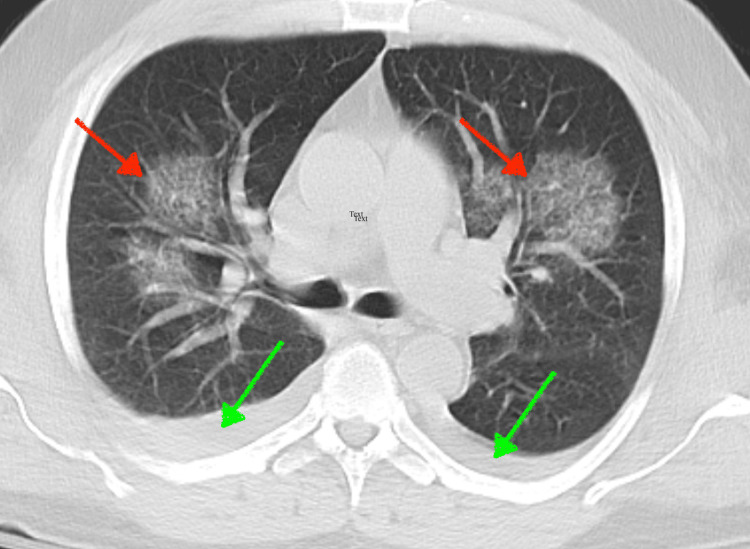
CT chest Lungs: Patchy groundglass opacities both upper lobes (red arrows). Small, bilateral pleural effusions with atelectatic change lung bases (green arrows). Enlarged subcarinal lymph gastroesophageal junction lymph nodes were identified (not shown).
